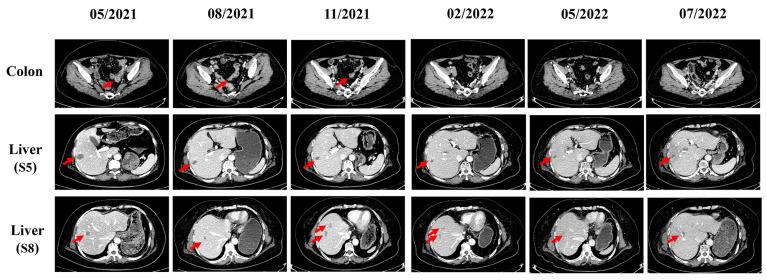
CT scans show the response of second-line and third-line therapies, including olaparib, a PD-1 Inhibitor, bevacizumab, and SBRT. The arrows refer to the location of the lesions (colon tumor and liver metastases). After combinational treatment involving olaparib with a PD-1 Inhibitor and bevacizumab, shrunken tumor was observed in August 2021 (colon tumor: length, 21 mm; liver metastases: S5, 20 mm × 15 mm; S8, 10 mm × 11 mm). In November 2021, the number of liver metastases increased. After combining SBRT, liver metastases gradually shrunk (S5, 17 mm × 14 mm; S8, 14 mm × 13 mm in July 2022).
